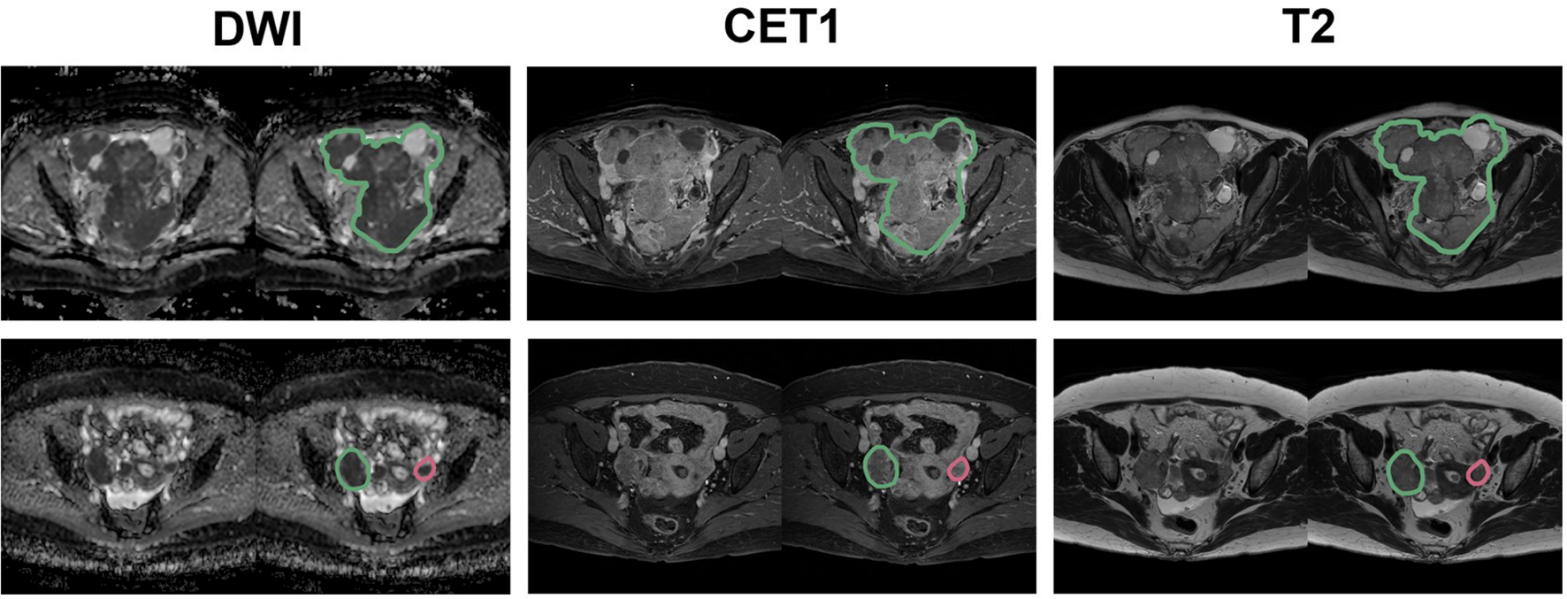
Representative placement of ROIs. The green contour indicates the largest tumor, while the red contour indicates the second-largest tumor for DWI, CET1, and T2 images.
